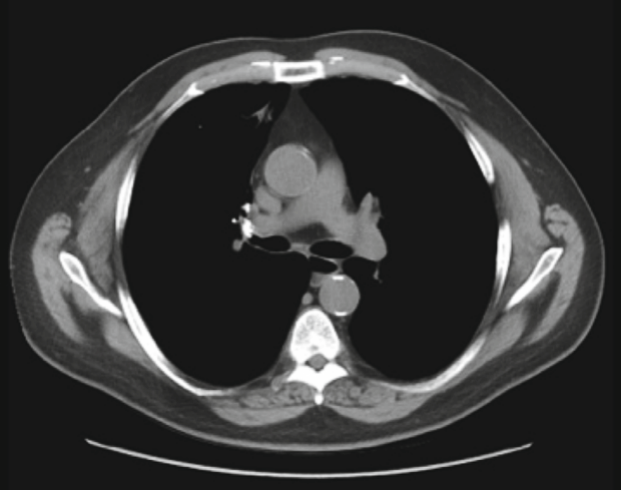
Axial image of computed tomography of the chest showing the suggestion of a broncholith in the anterior RUL bronchus.
